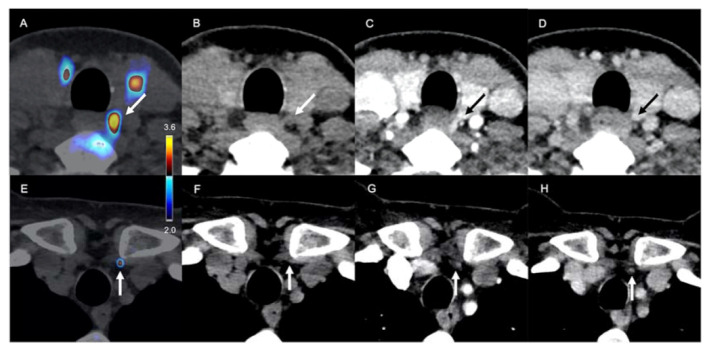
^18^F-FCH PET/CT and 4D-CT of a 56-year-old woman with recurrent pHPT and a history of upper and lower right parathyroidectomy (adenoma and hyperplasic gland, respectively). From left to right: fused PET/CT, non-contrast-enhanced CT, arterial-phase CT (30 s), venous-phase CT (70 s). Upper panel: lateral esophageal upper left adenoma (white and black arrows) with focal uptake of ^18^F-FCH (SUVmax = 4) (A), spontaneous density of 66 HU (B), high enhancement at the arterial phase (137 HU) (C), and washout > 20 HU at the venous phase (102 HU) (D). Lower panel: hyperfunctioning parathyroid in the left thyrothymic ligament (white arrows) with focal uptake of ^18^F-FCH (SUVmax = 3.2) (E), spontaneous density of 50 HU (F), no significant enhancement at the arterial phase (62 HU) (G) or venous phase (49 HU) (H).
